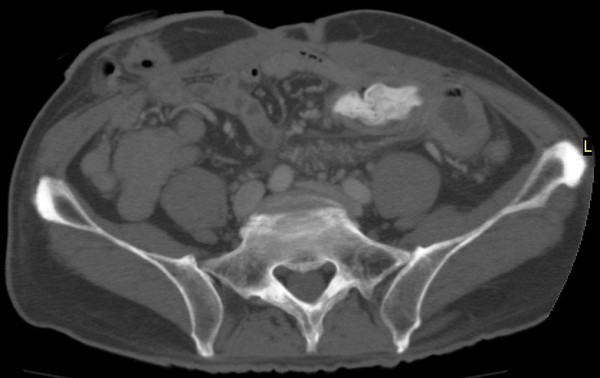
CT scan showed spacer migration into the abdominal cavity.
